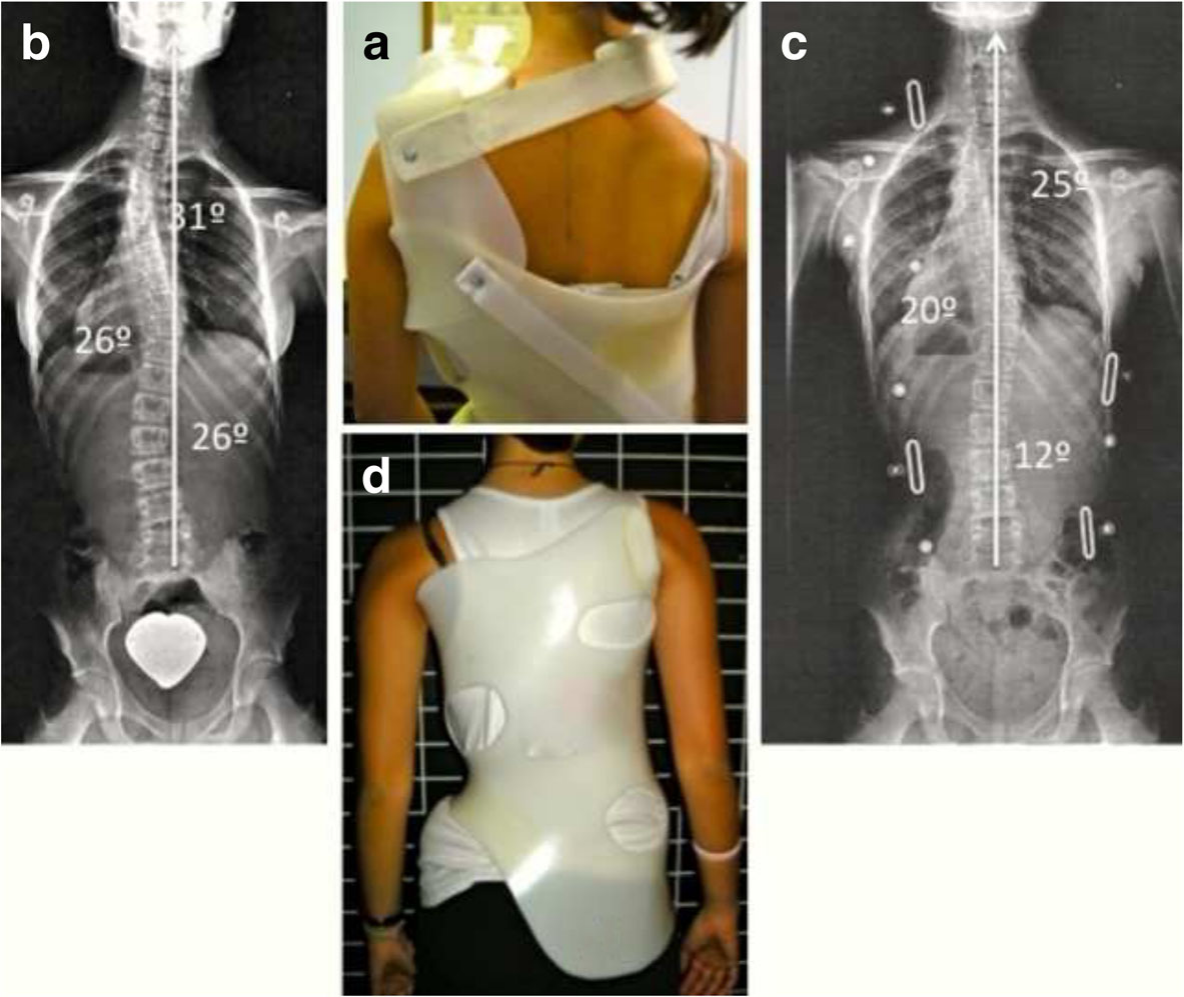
A girl with a primary triple structural curve (left proximal 31°, right main thoracic 26°, and left lumbar 26°) (b), was fitted with a first prototype including “D modifier” proximal pad in combination with a removable compression-traction superstructure (a). The in-brace X-ray showed a mild correction of all the curves (c). Spinal balance was significantly improved with this brace, and proximal curve did not increased but decreased also significantly. After observing that the apical region of the proximal curve was relatively low (disc T4–5), it was decided to design a complex brace, which included a third “three-point system” still working underarm (d) (no X-ray available). This exclusive design formed by three underarm “three-point systems” had not been used ever before, to our knowledge, and helped to stabilize this scoliosis. The risk of failure is the highest for this curve pattern, especially in this particular case where the proximal curve is the major and most rigid one
